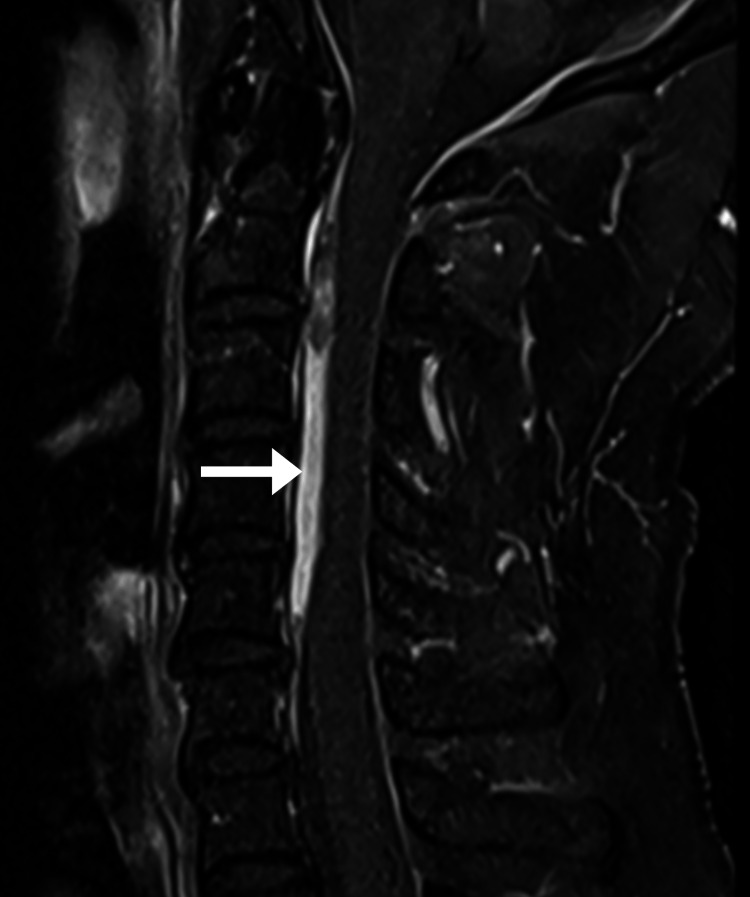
Cervical magnetic resonance imaging, mid-sagittal T1 fat-suppressed sequence with contrast The anterior epidural layer is re-demonstrated (arrow). However, there is no significant contrast enhancement noted, and no suspicious paraspinal soft tissue mass.
